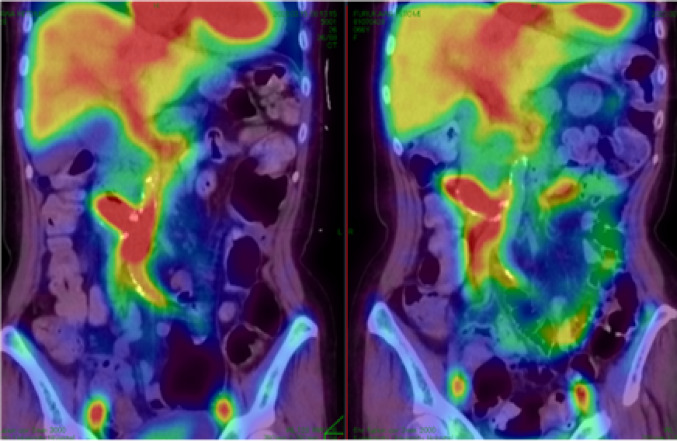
Bleeding scintigraphy. The images show bleeding in the jejunum that was suspected to originate from the duodenum. No obvious source of bleeding could be identified
